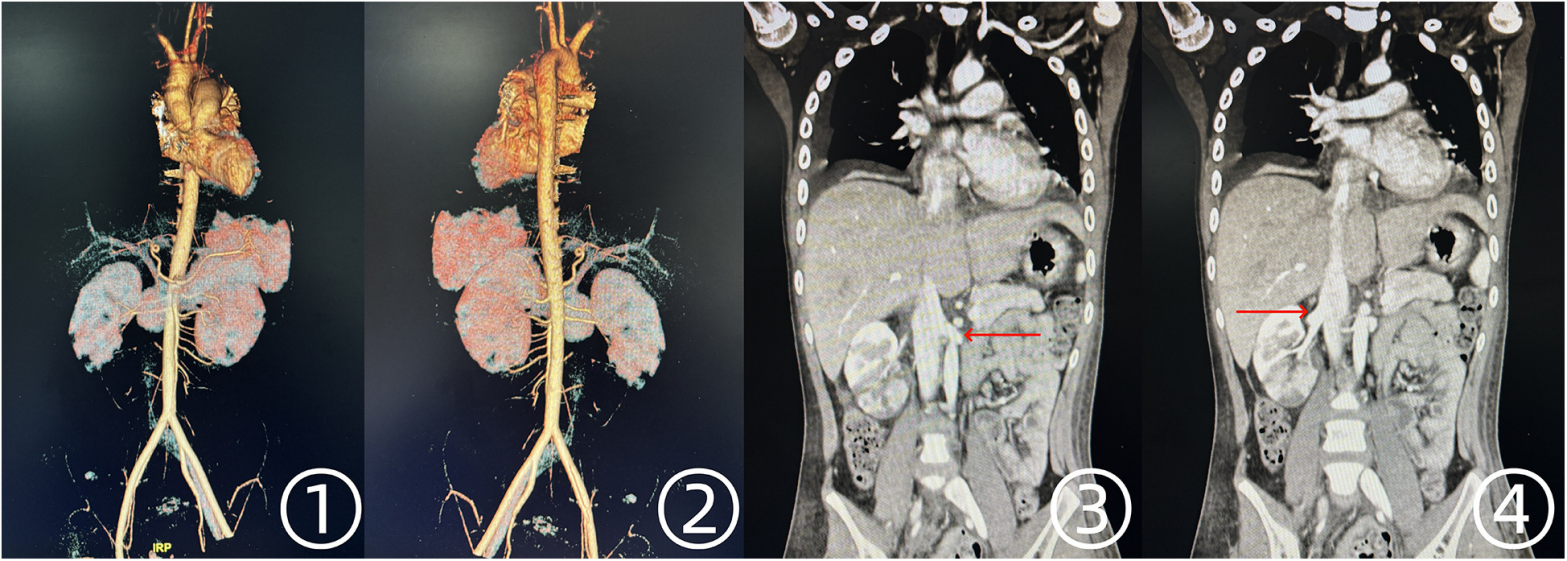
Postoperative CTA findings of the thoracic and abdominal aorta. ①, ② Unobstructed blood flow in the abdominal aorta and bilateral iliac arteries. ③, ④ Restored perfusion in both renal arteries.
